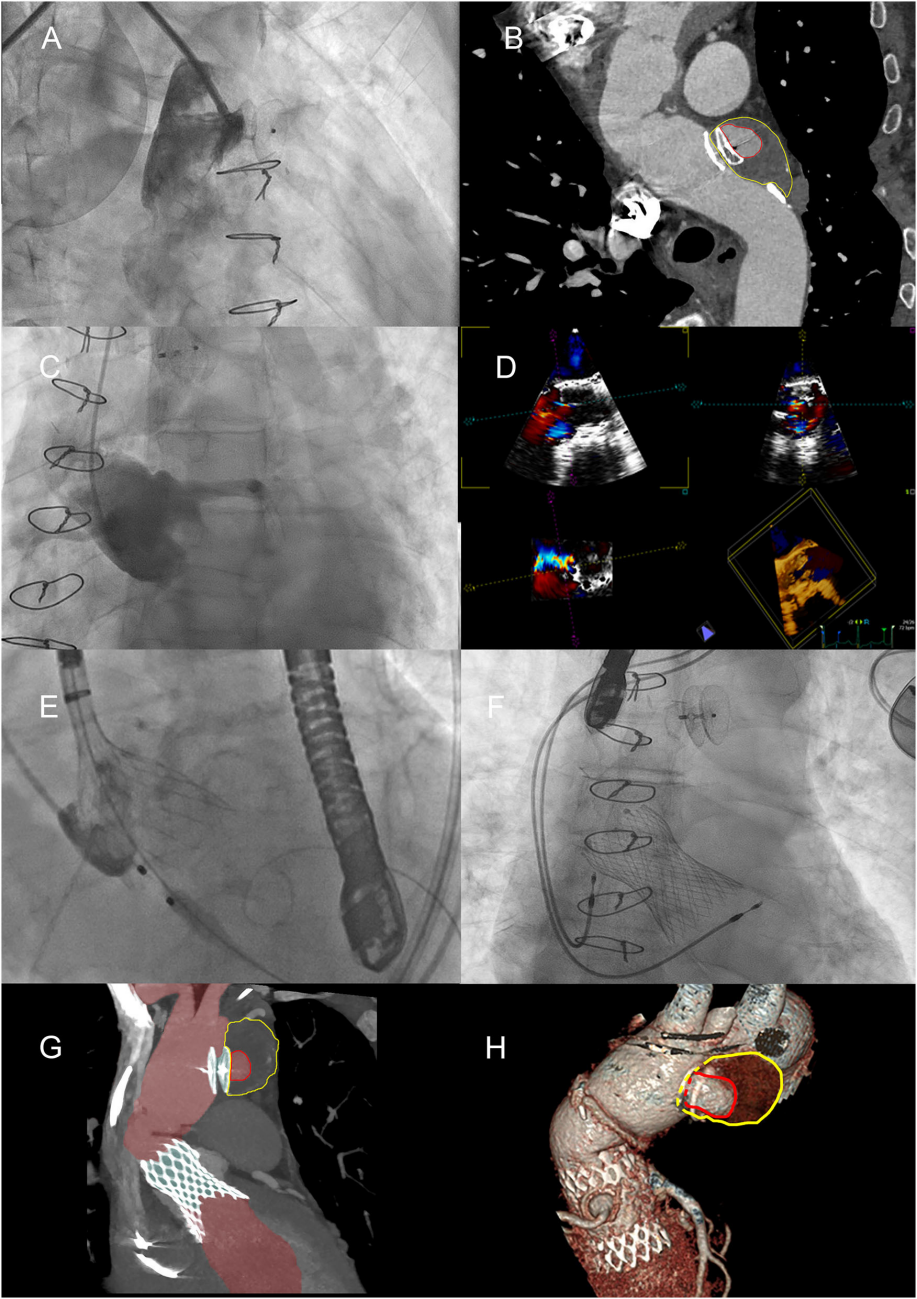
Positioning of the Amplatzer device and the Medtronic valve. (A) Positioning of the Amplatzer device, with angiography demonstrating the apparent total obliteration of the AAP. (B) MPR curved reconstruction of angio-CT, 1 month after placing the Amplatzer device. The red line highlights the perfused part of the AAP; the yellow line highlights the thrombosed part. (C) Severe failure of the native aortic valve, confirmed by angiography. (D) Study of the native aortic valve and confirmation of its severe insufficiency with transesophageal ultrasound. (E) Medtronic valve positioning by accurately locating the position of the annulus. (F) One-year later research for endoleak by transesophageal ultrasound. (G) Maximum intensity projection (MIP) reconstruction of angio-CT after placement of TAVI. The perfused part of the aorta and AAP is highlighted in red. (H) VR reconstructions of the partially thrombosed AAP.
